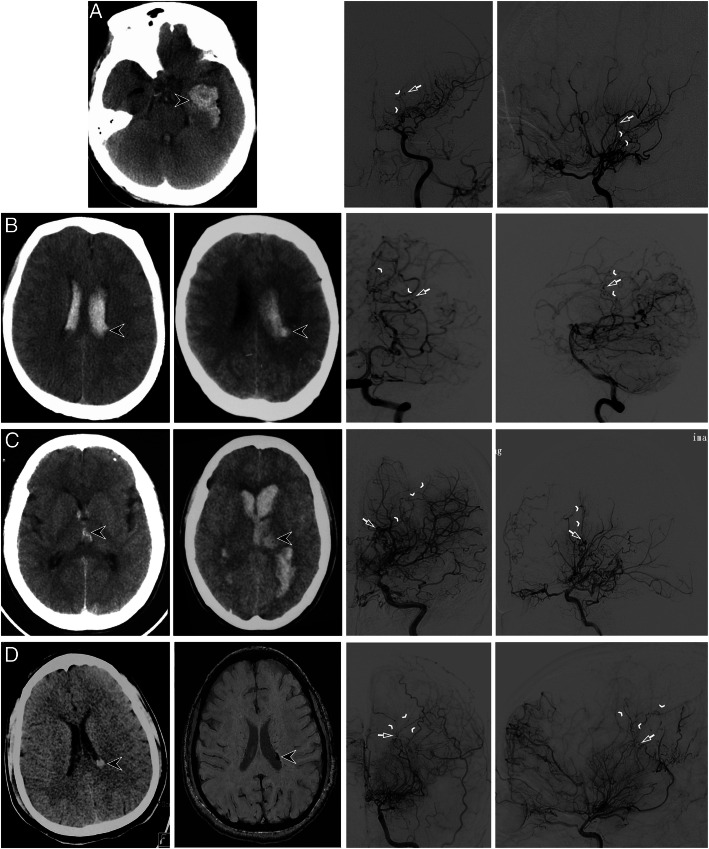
Representative cases of target anastomotic territory patterns. Hemorrhagic sites during the acute phase are indicated by black arrowheads on noncontrast-enhanced CT scans. Target collateral vessels are indicated by white arrowheads and hemorrhagic sites are indicated by white arrows on angiograms. a: A 58-year-old man experienced an intraventricular hemorrhage and was treated with conservative therapy. A CT image *(left)* indicates the initial hemorrhagic site located at the left temporal horn of the lateral ventricle. Left anterior-posterior *(middle)* and lateral *(right)* carotid artery angiograms obtained at baseline demonstrate the anterior choroidal artery responsible for the hemorrhage. No obvious medullary artery anastomosis could be observed. After > 70 months of clinical follow-up, no recurrent hemorrhage had occurred. b: A 50-year-old man experienced a recurrent hemorrhage in the ipsilateral hemisphere. A CT image *(left)* indicates the initial hemorrhage in the posterior portion of the body of the left lateral ventricle. A CT image obtained 49 months later *(second from left)* demonstrates a recurrent hemorrhage in the initial hemorrhagic site. Anterior-posterior (*second from right*) and lateral (*right*) views of the left vertebral angiogram obtained at baseline reveal the lateral posterior choroidal artery responsible for the initial and recurrent hemorrhages. The angiograms demonstrate obvious medial medullary artery anastomosis between the target collateral vessel and the territory fed by the paracentral artery. (Modified with permission from Wang et al. *AJNR Am J Neuroradiol*. 2019;40(10):1665–1671.^4^). c: A 36-year-old woman experienced a recurrent hemorrhage in the ipsilateral hemisphere. A CT image *(left)* demonstrates the initial hemorrhage in the body of the left lateral ventricle. A CT image obtained 49 months later *(second from left)* demonstrates a recurrent hemorrhage near the initial hemorrhagic site. Anterior-posterior (*second from right*) and lateral (*right*) views of the left carotid angiogram obtained at baseline reveal the lenticulostriate artery anastomosis responsible for the initial and recurrent hemorrhages. The angiograms demonstrate obvious lateral medullary artery anastomosis between the target collateral vessel and the central artery territory. d: A 36-year-old man experienced an intraventricular hemorrhage and was treated with conservative therapy. CT images (confirmed by susceptibility-weighted imaging) *(left and second from left)* indicate the initial hemorrhagic site located at the left posterior body of the lateral ventricle. Left anterior-posterior *(second from right)* and lateral *(right)* carotid artery angiograms obtained at baseline demonstrate the anterior choroidal artery responsible for the hemorrhage. The angiograms demonstrate obvious multiple medullary artery anastomosis between the target collateral vessel and the anterior parietal artery, paracentral artery, and angular gyrus artery territory. After > 96 months of clinical follow-up, no recurrent hemorrhage had occurred
